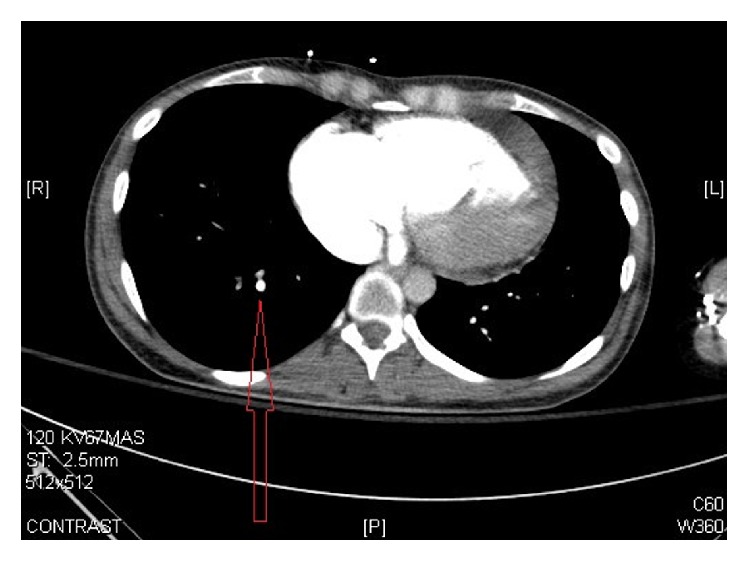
CT scan with contrast of the chest showing small distal right pulmonary embolus.
